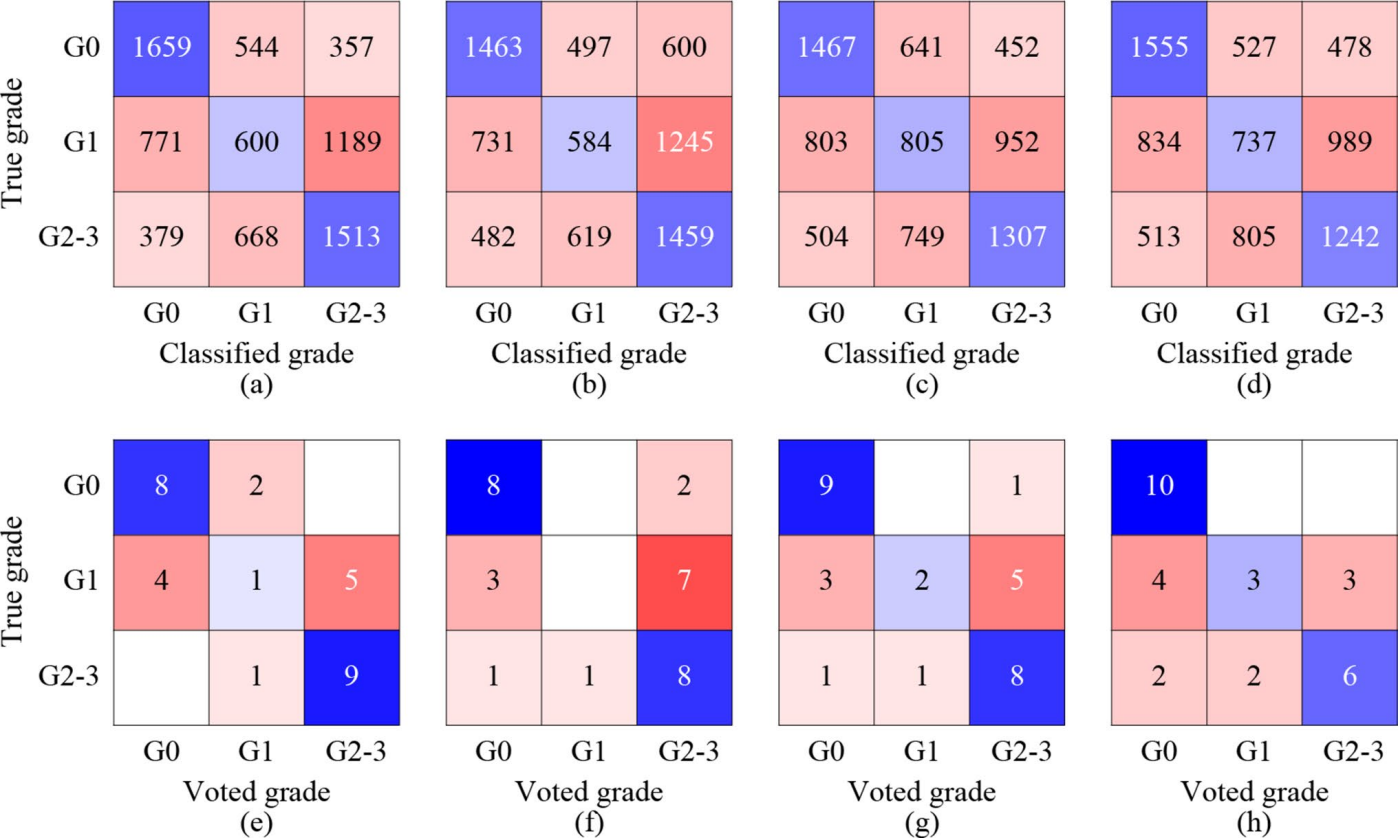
Classification results of the ROIs of a ultrasound images, b first-order moment maps, c fourth-order moment maps, d moment heatmaps obtained by the CNN, and those of cases obtained through a hard voting on the classified ROIs of e ultrasound images, f first-order moment maps, g fourth-order moment maps, and h moment heatmaps
